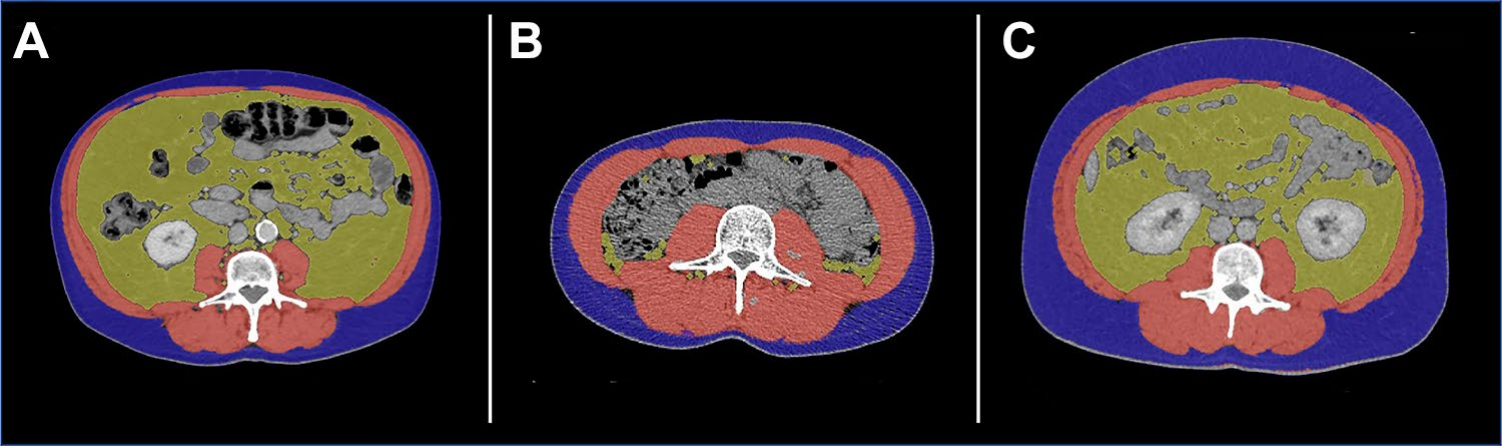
Representative CT scans assessed using CoreSlicer with the following body parameters highlighted: visceral fat (VFA, yellow), skeletal muscle (red), subcutaneous fat (SFA, blue). Examples of patients with A high VFA, low SFA; B low VFA, low SFA; C high VFA, high SFA
